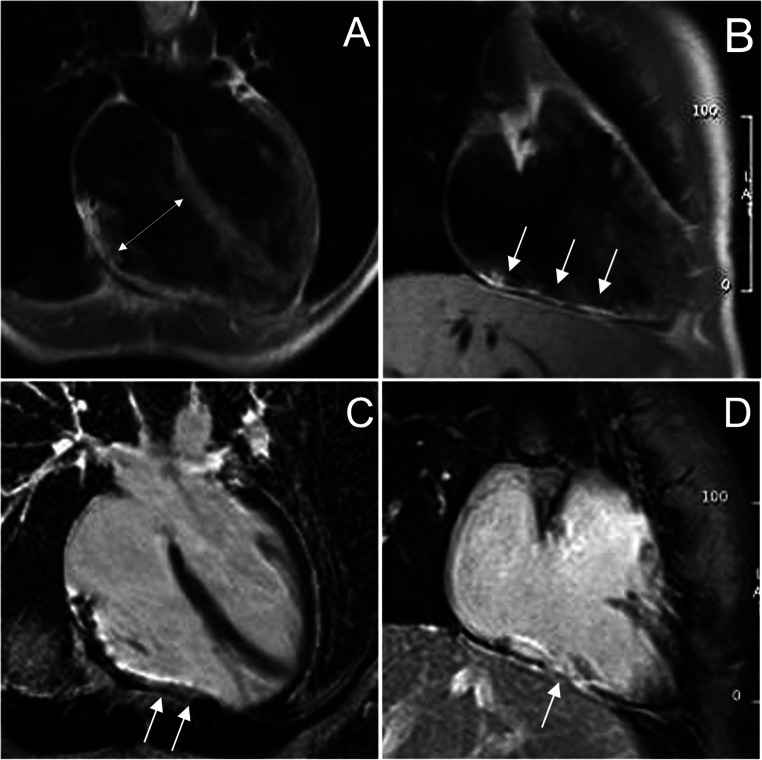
CMR images in a patient with a right dominant ACM. T1-weighted (A, B) and post-contrast images (C, D) showing the dilatation (A, arrow) and the fibro-fatty infiltration (arrows, B, C, D) of the free and diaphragmatic wall of the RV. ACM, arrhythmogenic cardiomyopathy; RV, right ventricle
